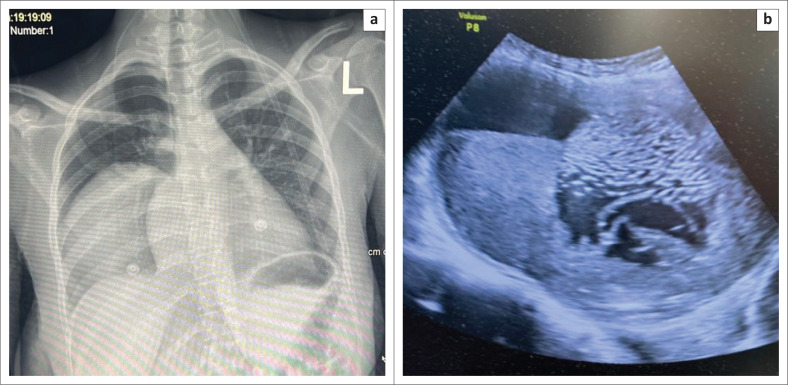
(a) Chest X-ray displaying right sided pulmonary cystic lesion. (b) Sagittal ultrasound view of the uterus demonstrating a cystic lesion with septation and a daughter cyst.
